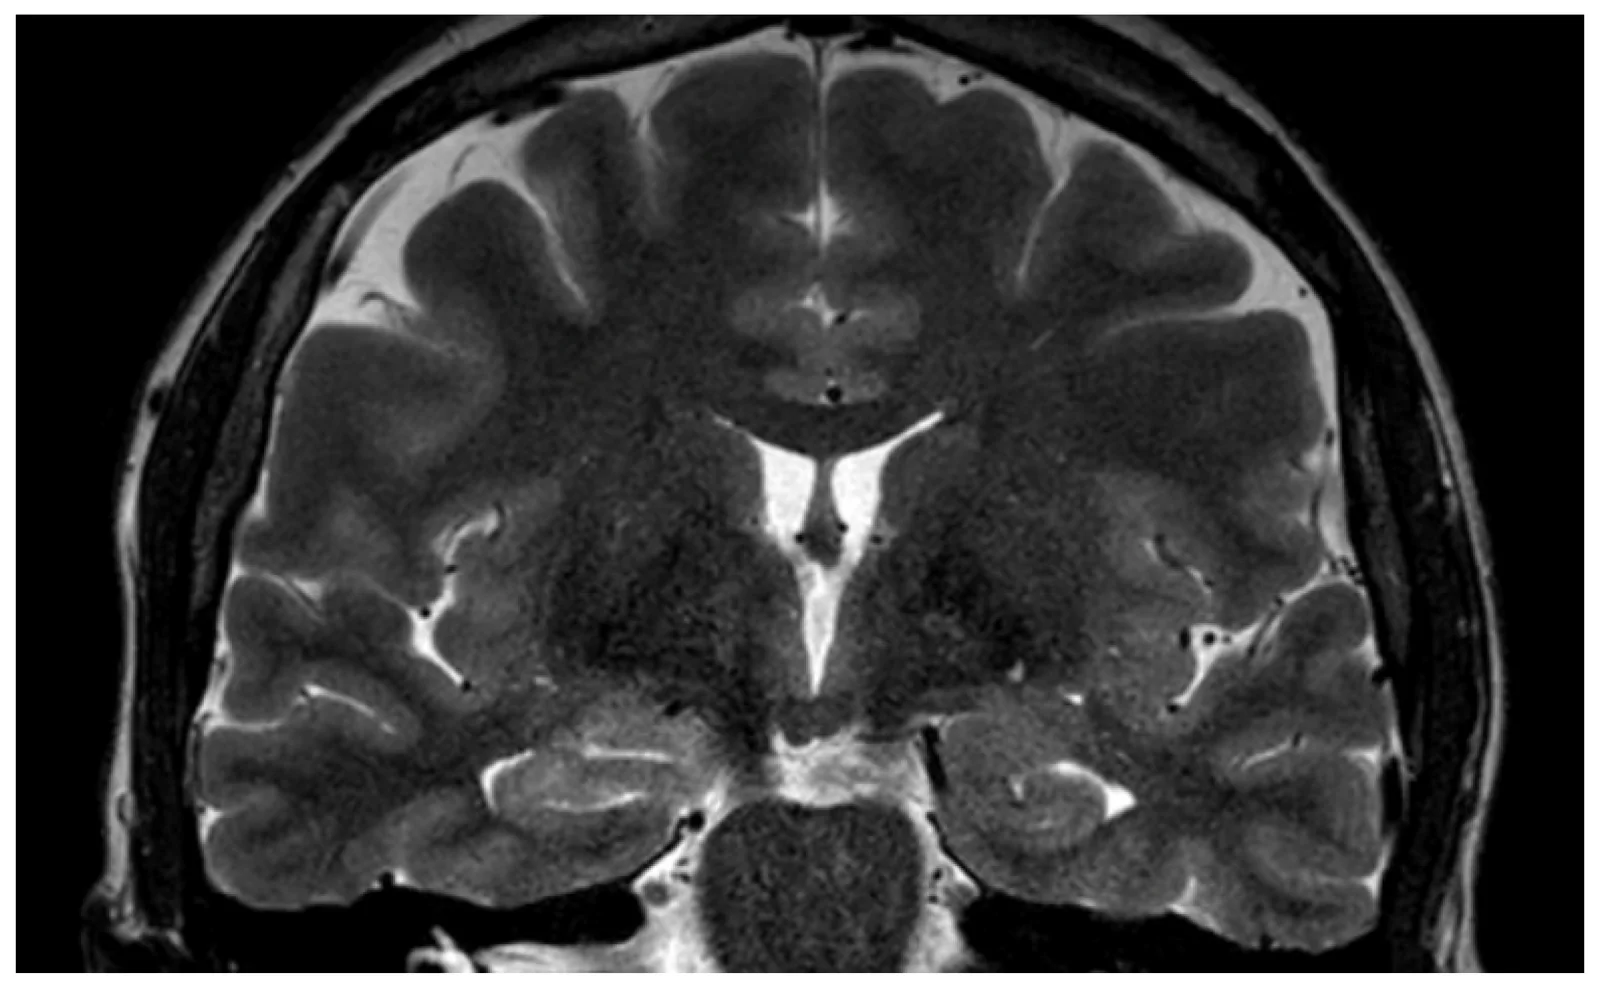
Coronal T2-weighted MRI through the medial temporal lobes demonstrating interval regression of the previously described right hippocampal lesion, with no clearly demarcated residual hyperintense focus visible on this sequence.
